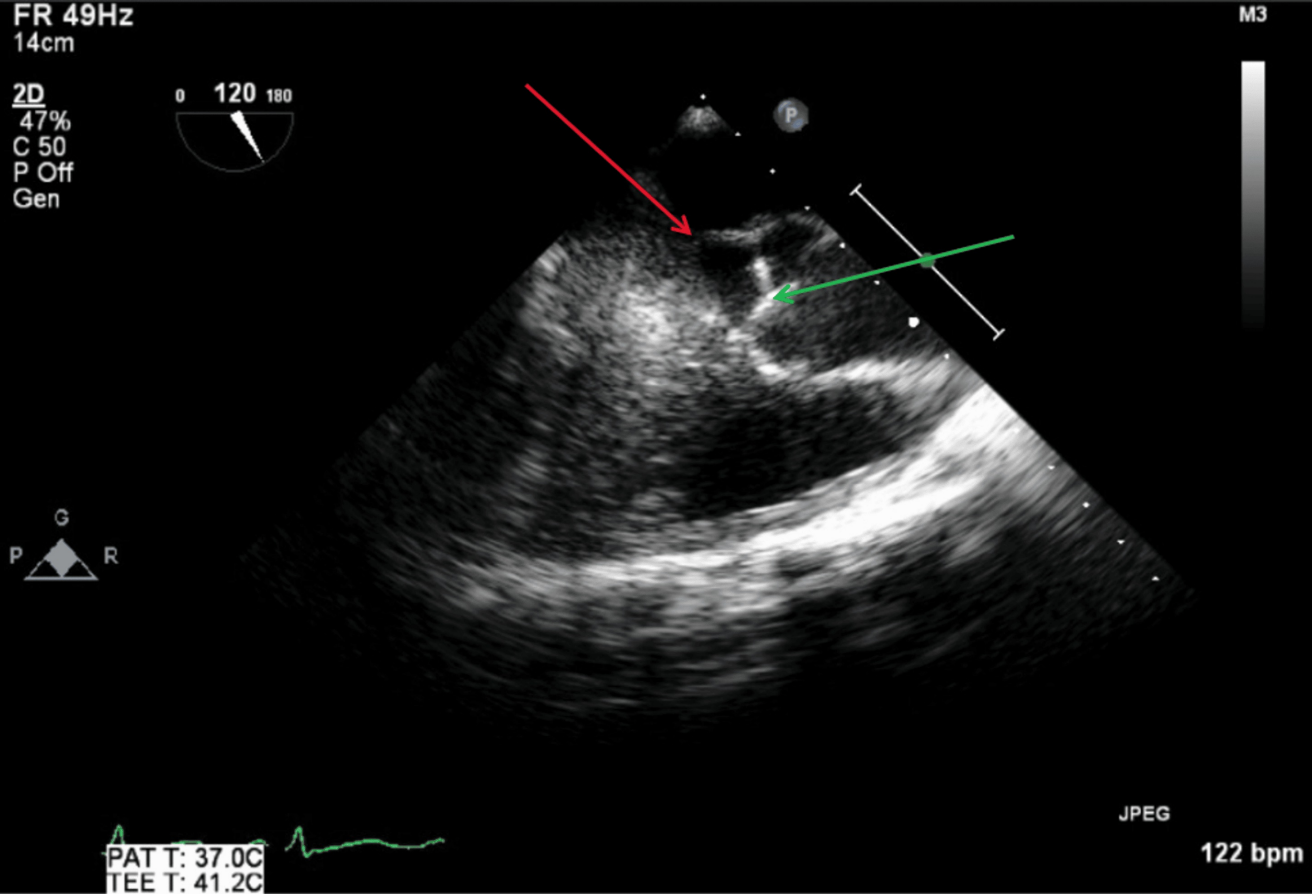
TEE mid-esophageal long-axis view during systole. The red arrow shows mitral valve with no visible vegetations. The green arrow shows aortic valve with no visible vegetations. TEE: transesophageal echocardiography
